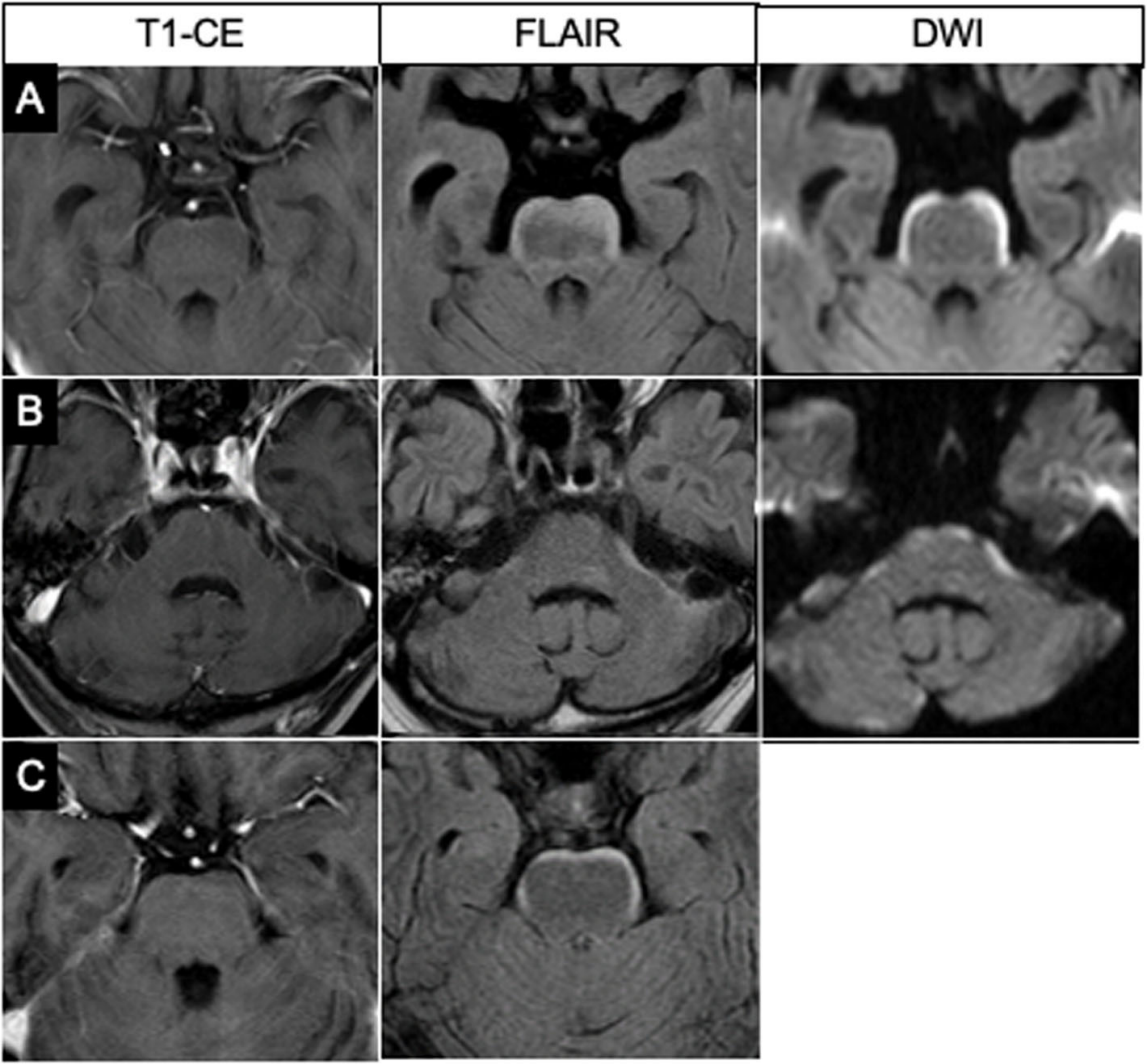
Three representative cases of bloomy rind sign. Although no abnormalities are seen on the brainstem on GdCE T1-weighted images, FLAIR images show hyperintensity along the brainstem surface in all three cases. Figure 2a and c show FLAIR hyperintensity on the antero-lateral surface of the pons, and Fig. 2b shows it along the antero-lateral cerebellar peduncle. DW images show restricted diffusion at the same lesions of two patients. DW images are not available for the third patient. Two patients (Figs. a and c) had positive CSF cytology, but one (Fig. b) did not undergo CSF examination because of neurological signs and restricted enhancement in the internal acoustic canals on GdCE images
